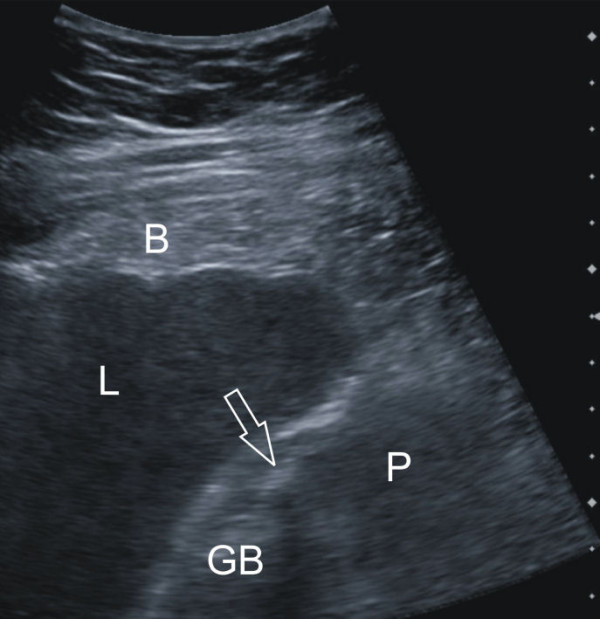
Sonography of the abdomen. This was performed after admission to our surgical department. Because of the lack of dorsal ultrasound reinforcement, the mass (P) surrounding the gallbladder (GB) was considered to be blood, pus or less likely tumorous soft tissue, not ascites. The transparent arrow indicates a stone.
